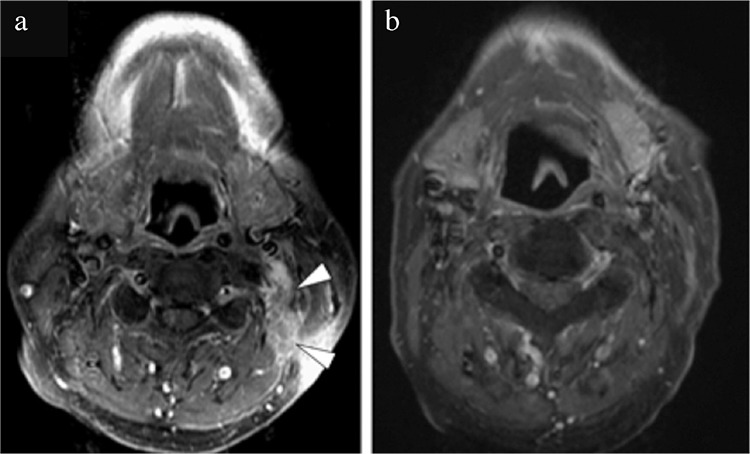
Magnetic resonance imaging finding 3 months after resection (a). A small residual tumor was seen locally above the scalenus medius muscle (white arrow head). The latest magnetic resonance imaging finding (b). No evidence of disease was observed 7 years after radiotherapy.
